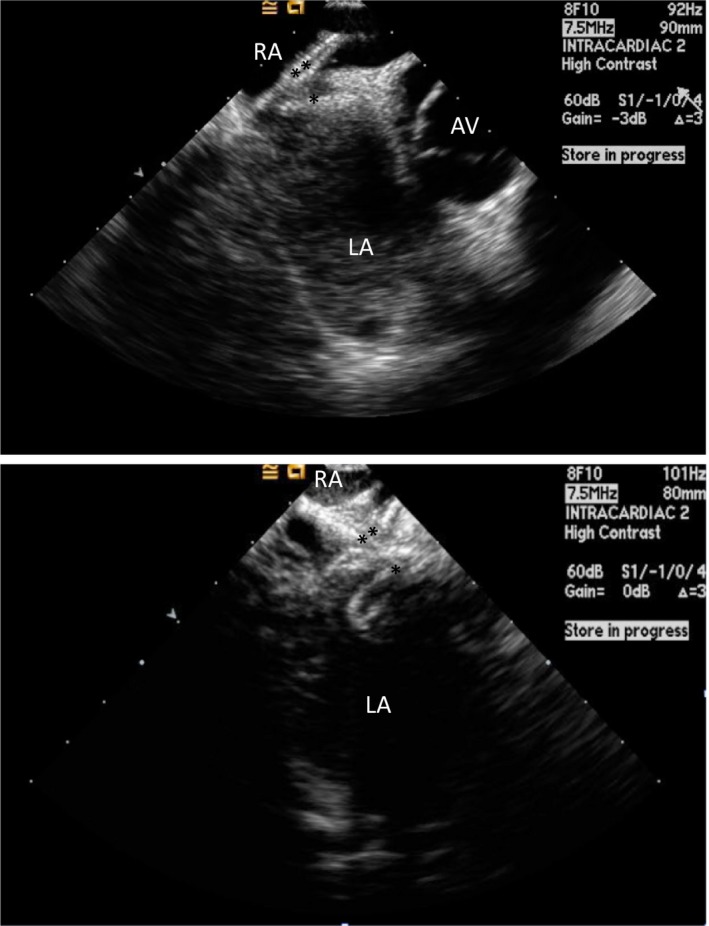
Intracardiac echocardiogram image of the right () and left atrial disc (*) of an Amplatzer PFO Occluder (top) and Gore Cardioform Septal Occluder (bottom) before the release of the device. Note the splaying of the discs at the aortic rim. AV indicates aortic valve; LA, left atrium; RA, right atrium.
